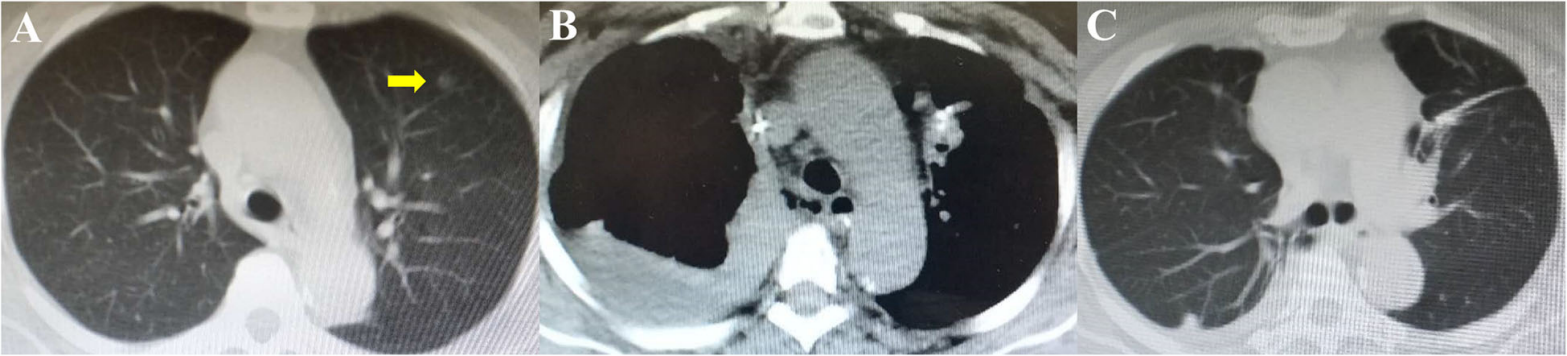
The computed tomography images. a A nodule in the left upper lobe was indicated by arrow. b The right-sided hydropneumothorax. c The esophagus was healed
